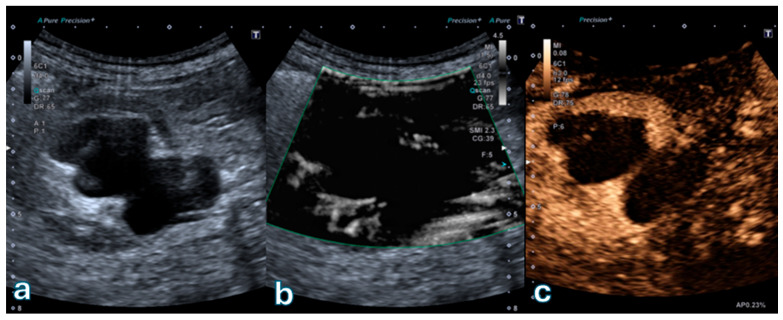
Complex renal cyst classified as Bosniak III. (a) B-mode ultrasound shows a multiloculated cystic lesion with thick, irregular walls. (b) Superb Microvascular Imaging (SMI) reveals intense microvascular signals along the thickened septa and walls, indicating marked vascularization. (c) Contrast-enhanced ultrasound (CEUS) demonstrates clear enhancement of the thick walls without a solid mural nodule, consistent with a Bosniak III indeterminate high-risk cystic lesion.
